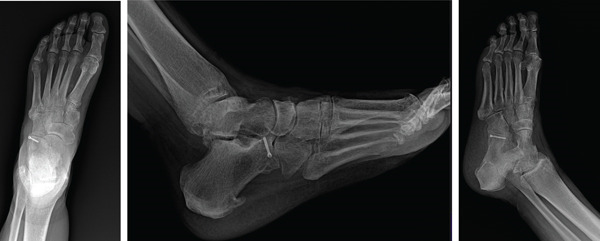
Anteroposterior, lateral, and oblique radiographs of left foot 8 months post‐op.
